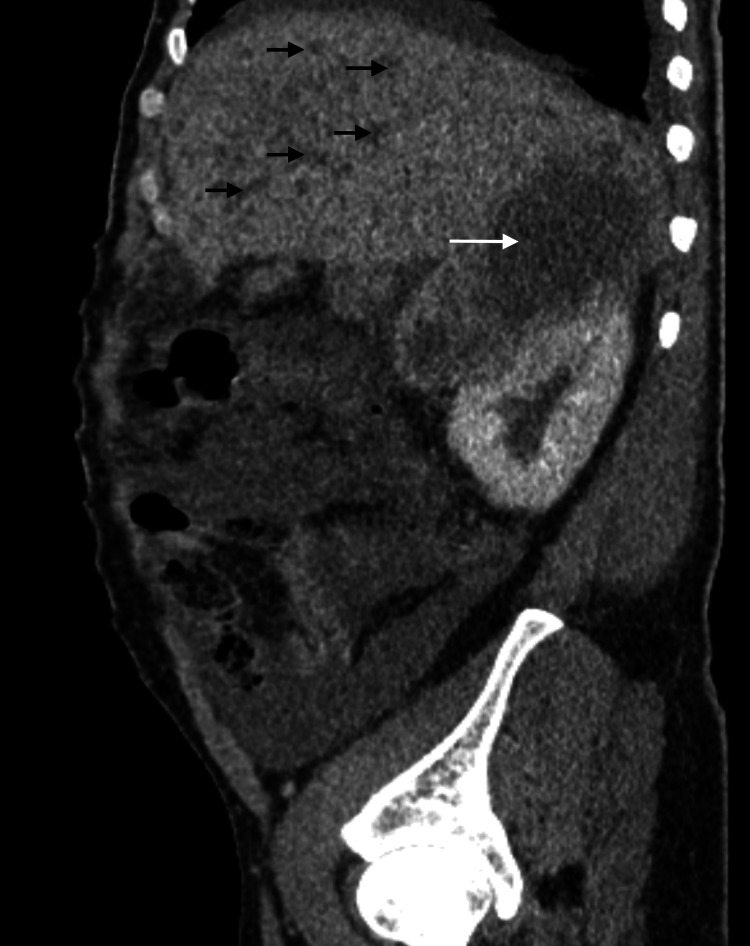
CT imaging showing multiple hypodense hepatic nodules (black arrows) and a large right adrenal mass (white arrow) measuring 14.6 x 9.4 x 9.3 cm in size
